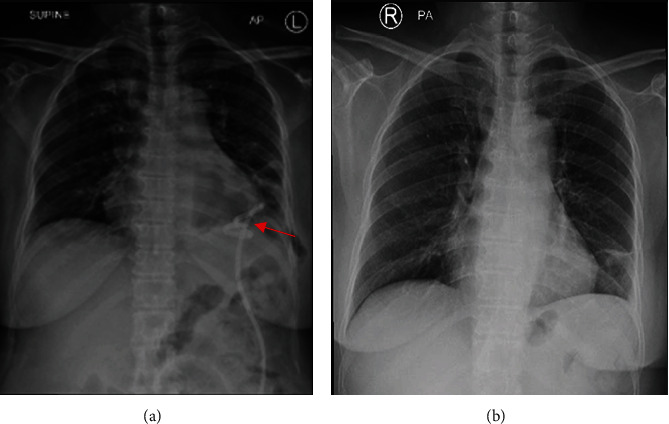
Chest X-ray postinsertion and predischarge. (a) Postinsertion of pigtail catheter in the left thoracic cavity (Red arrow). (b) Chest X-ray showing complete resolution of empyema and remarkable expansion of the left lower lobe.
